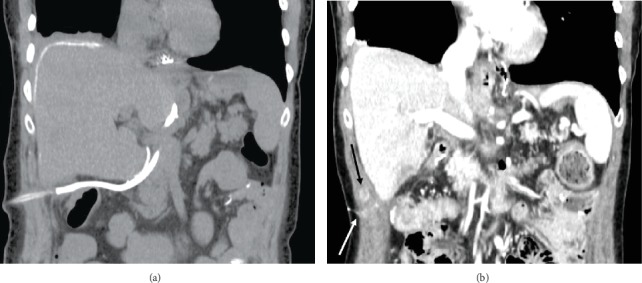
Coronal noncontrast (a) and postcontrast (b) CT reconstructions demonstrating a new vascular nodule (black arrow) in close proximity to the drain tract with the residual barium demarcating tract (white arrow).
